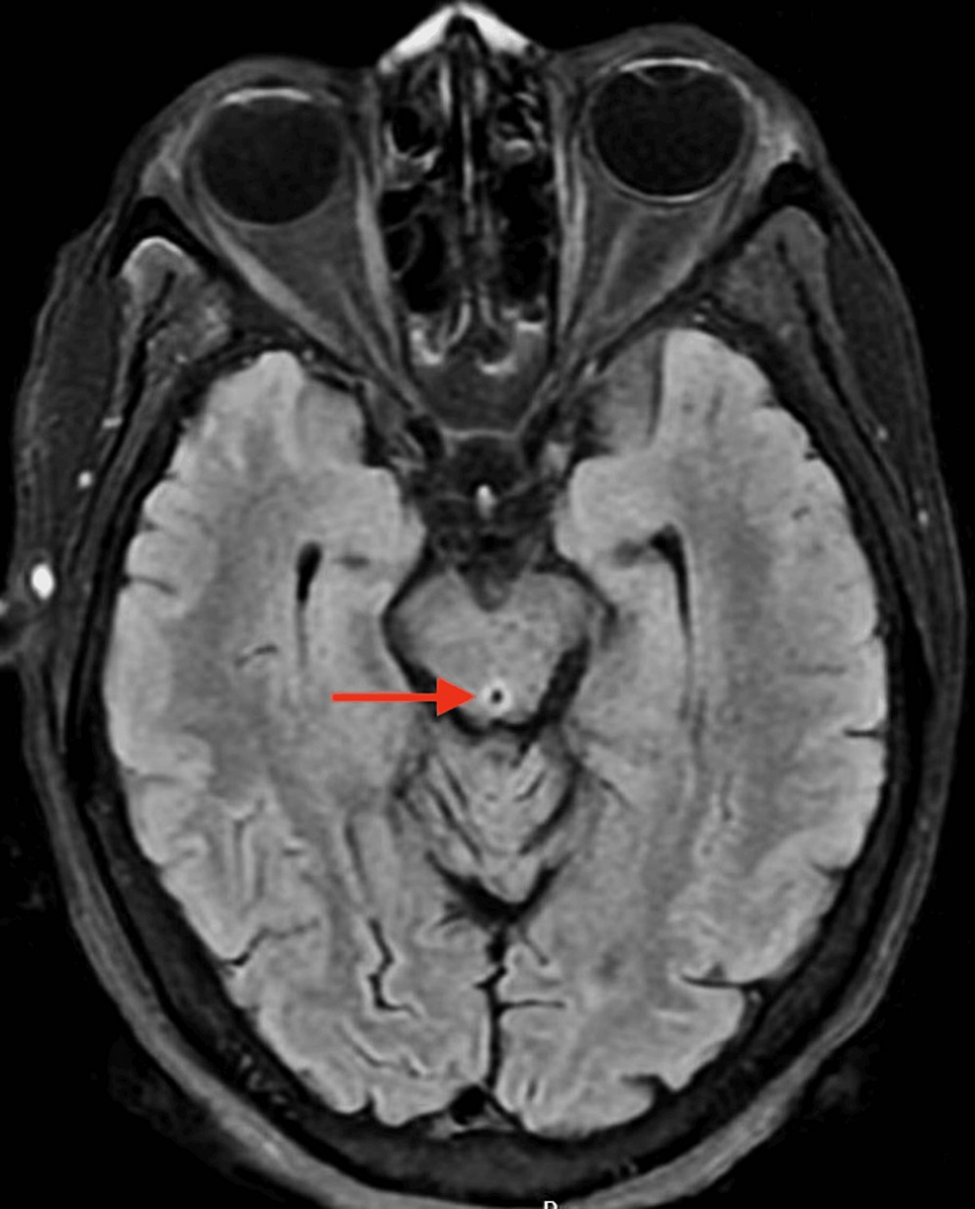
Non-contrast MRI, axial view demonstrating an increased T2 FLAIR signal in the periaqueductal grey matter. FLAIR: Fluid-attenuated inversion recovery
